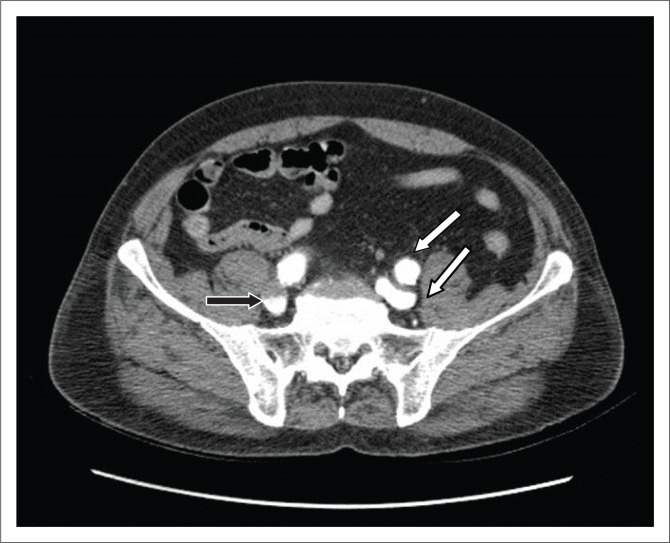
Axial contrast-enhanced computed tomography scan during the arterial phase showing opacification of the common iliac arteries and veins bilaterally, with similar attenuation (white arrows); layering of contrast was observed in the iliac veins (black arrow).
